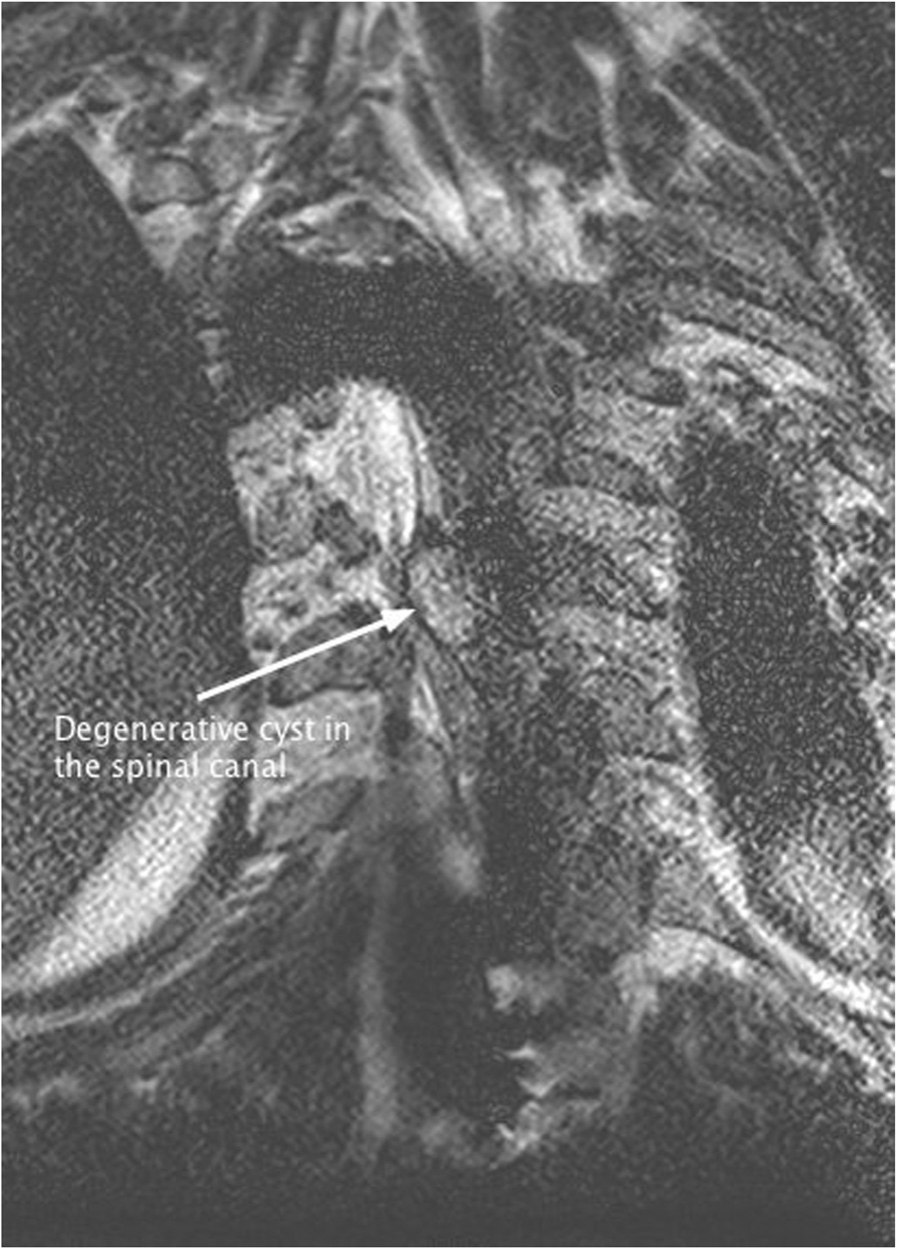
A coronal magnetic resonance image of the location of the cyst relative to the signal void caused by the proximal hook.
